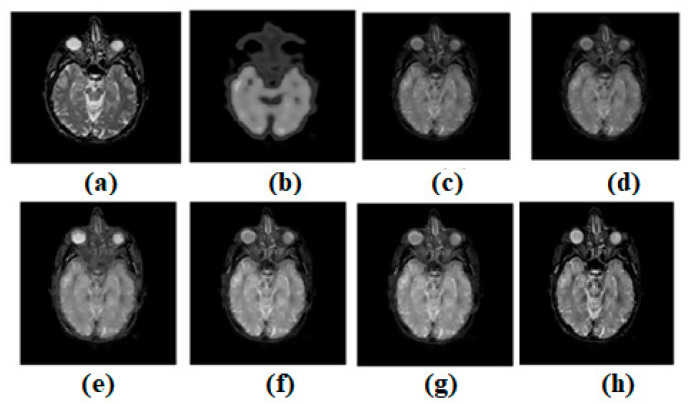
(a) Source image: MR-T2, (b) Source Image: SPECT, (c) Result of [44], (d) Result of [47], (e) Result of [48], (f) Result of [49], (g) Result of [51], (h) Result of [53].
